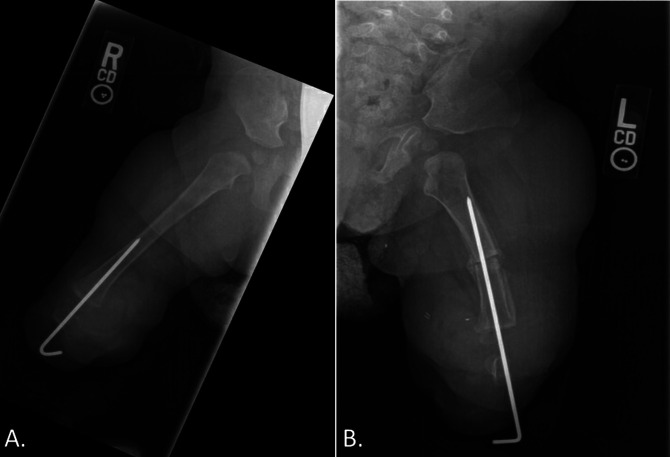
Radiographic anterior-posterior imaging of bilateral lower extremities at 1 month after procedure. A, Right lower extremity image demonstrating good alignment of the calcaneus and femur with bony healing. B, Left lower extremity image demonstrating a midshaft femur osteotomy with callus formation and appropriate alignment of the calcaneus and femur.
